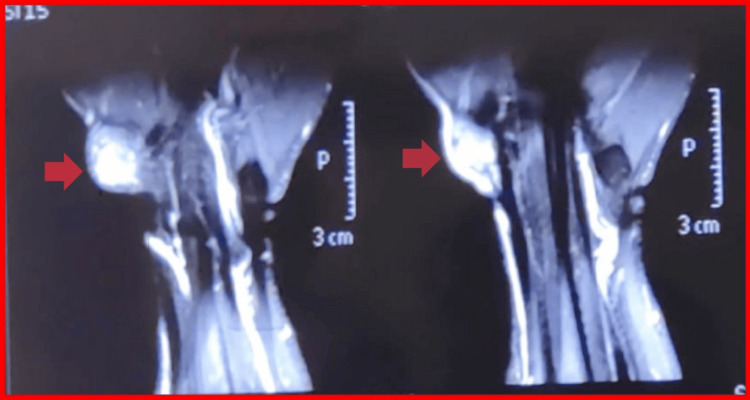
T2-weighted coronal images of the wrist The TGCT is hyperintense on this coronal MRI section, marked by a red arrowhead, with the radial artery appearing as a hyperintense vessel pushed laterally by the tumor. A blooming artifact due to hemosiderin deposits was also observed. TGCT: tenosynovial giant cell tumor; MRI: magnetic resonance imaging
